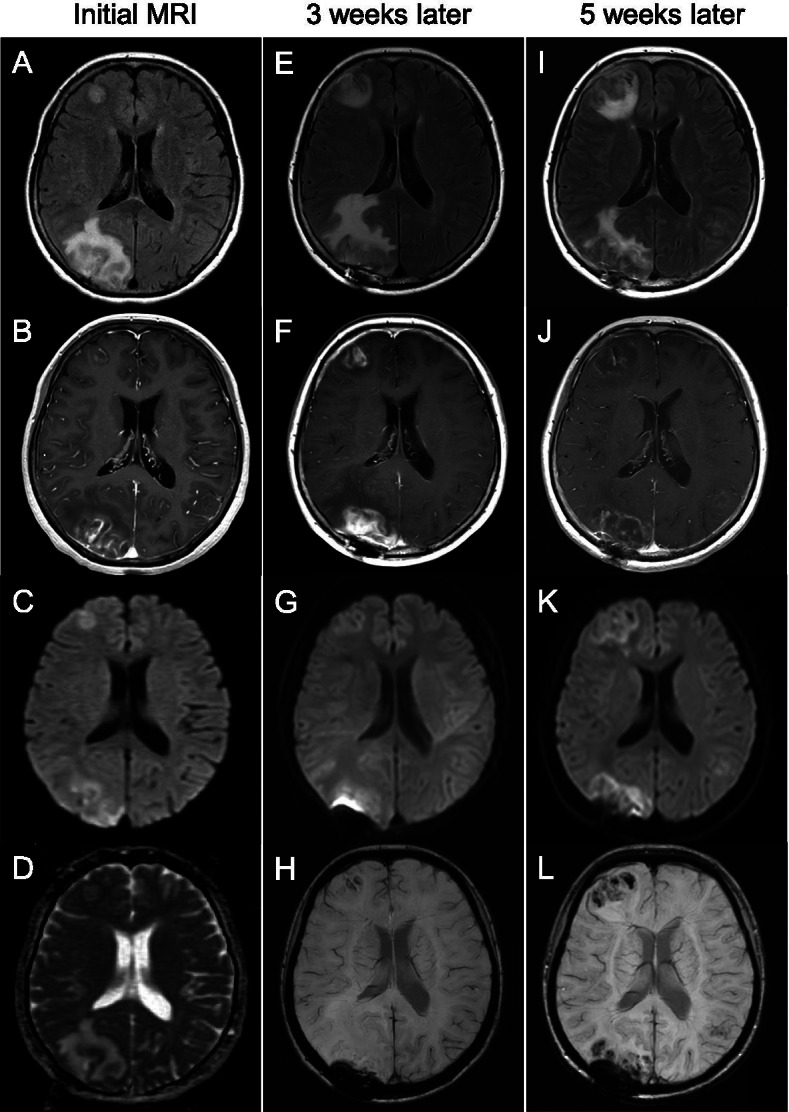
Neuroradiologic MRI findings. Magnetic resonance imaging (MRI) of the brain performed at the time of admission (A–D) and 3 weeks (E–H) and 5 weeks after (I–L) the initial MRI. Axial fluid-attenuated inversion recovery (FLAIR) (A, E, and I), gadolinium-enhanced T1-weighted image (Gd-T1WI) (B, F, and J), diffusion-weighted image (DWI) (C, G, and K), and susceptibility-weighted image (SWI) (H and L) showed multiple progressive nodules with hemorrhagic and necrotic lesions in the right frontal lobe, right occipital lobe, and left parietal lobe (not shown). Apparent diffusion coefficient (ADC) map on admission showed low signal intensity in the center areas and high signal intensity in the surrounding areas in the right occipital lobe lesion (D). The biopsied scar was found in the right occipital lobe (E–L)
